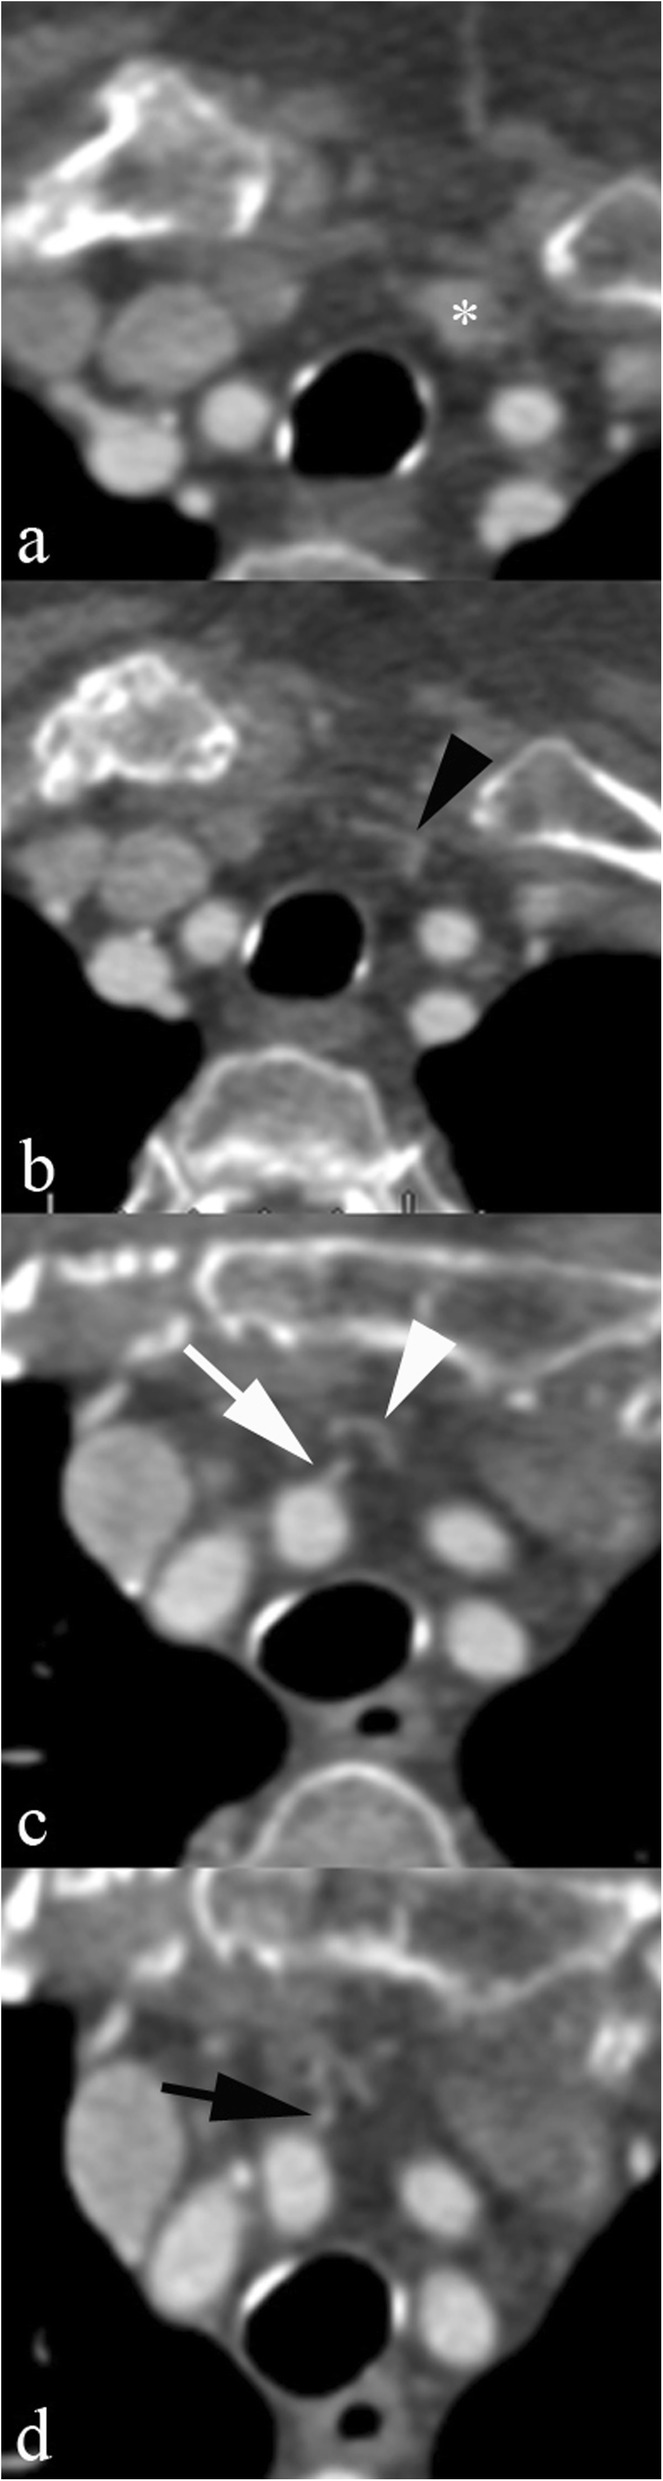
Thyroid ima artery. Axial CT scan: a thin artery arises from the right common carotid artery (arrow in c), bends inferiorly (arrow in d) and then turns upwards (arrowheads in b and c) to reach the lower pole of the left thyroid gland lobe (asterisk in a)
